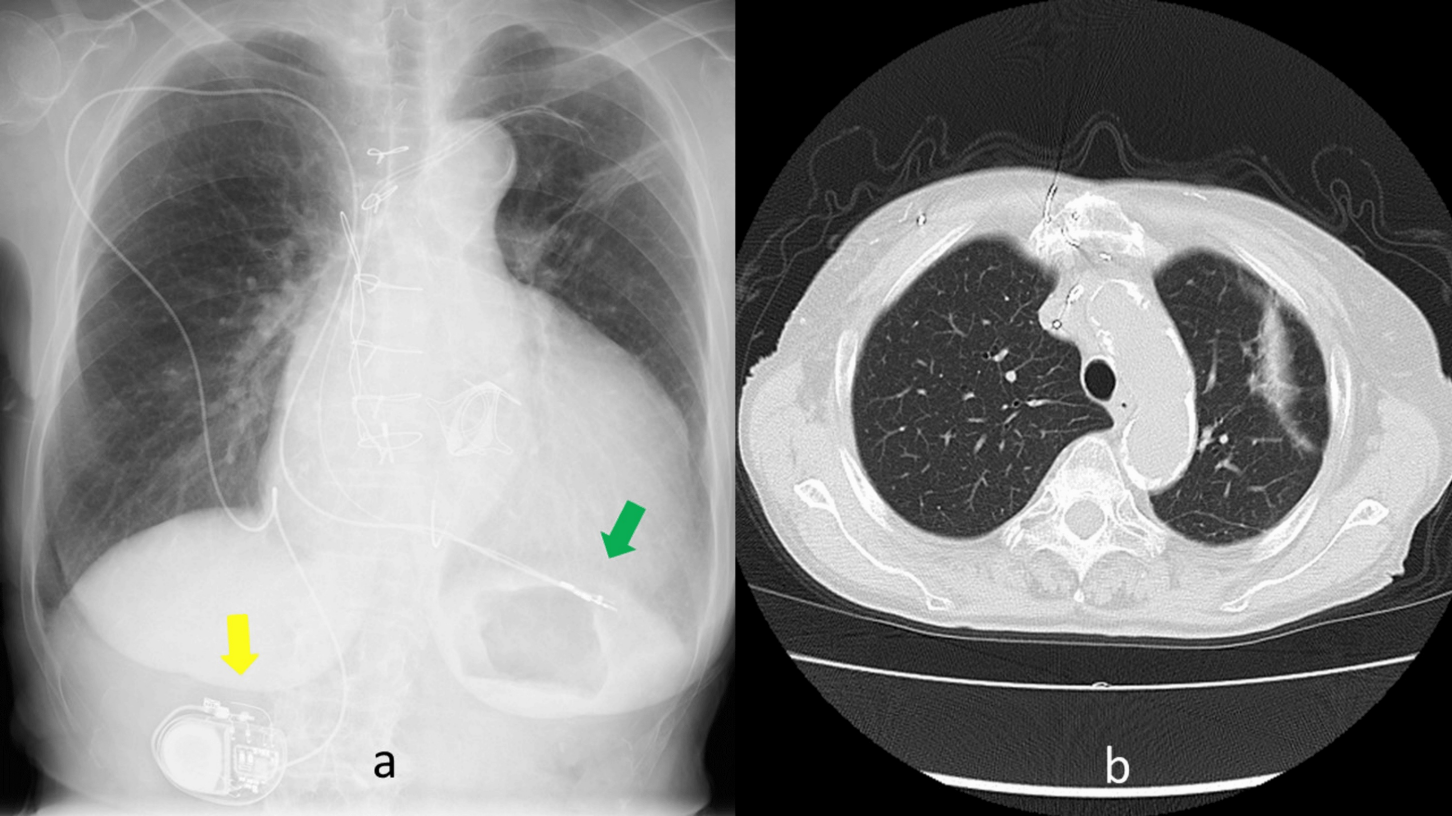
Chest X-ray film and CT scan. (a) The yellow arrow indicates the translocated pacemaker. The green arrow indicates the tip of the pacing lead. Consolidation can be seen in the left upper lobe. (b) CT at four years and two months after radiation therapy. Only scar exists.
